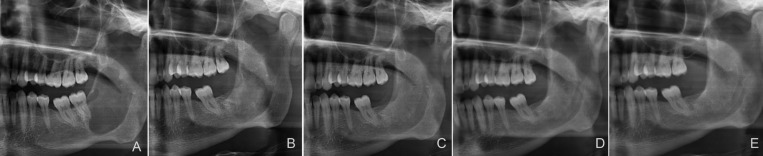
(A) panoramic radiograph of odontogenic keratocyst before decompression; (B) panoramic radiograph 1 year after decompression and before subsequent enucleation; (C) panoramic radiograph 1 year after subsequent enucleation; (D) panoramic radiograph obtained 2 years after subsequent enucleation; (E) panoramic radiograph 5 years after subsequent enucleation.
